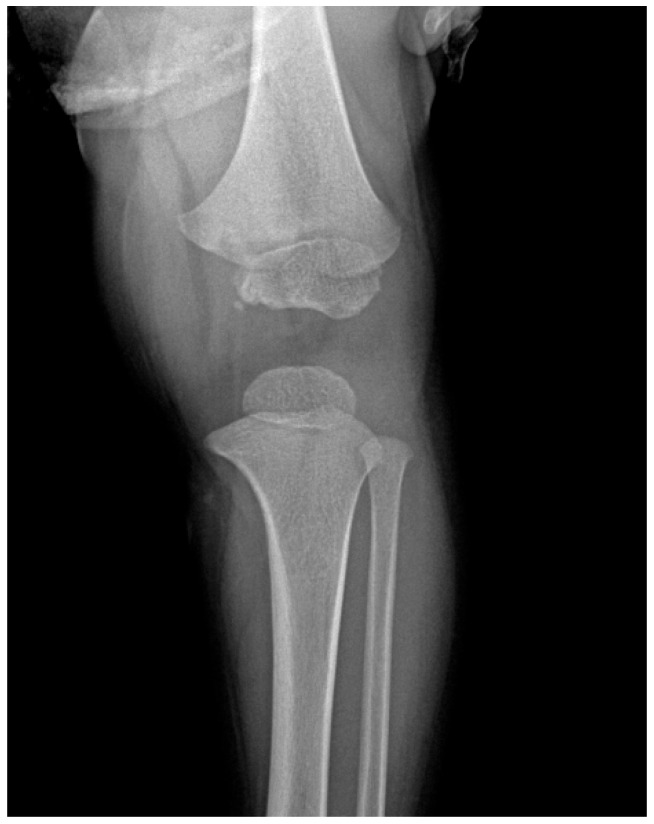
X-ray of the left knee reveals mixed osteocondensation and osteolytic areas in the distal third of the left femur. Additionally, there is evidence of soft tissue inflammation medial to the distal end of the left femur.
